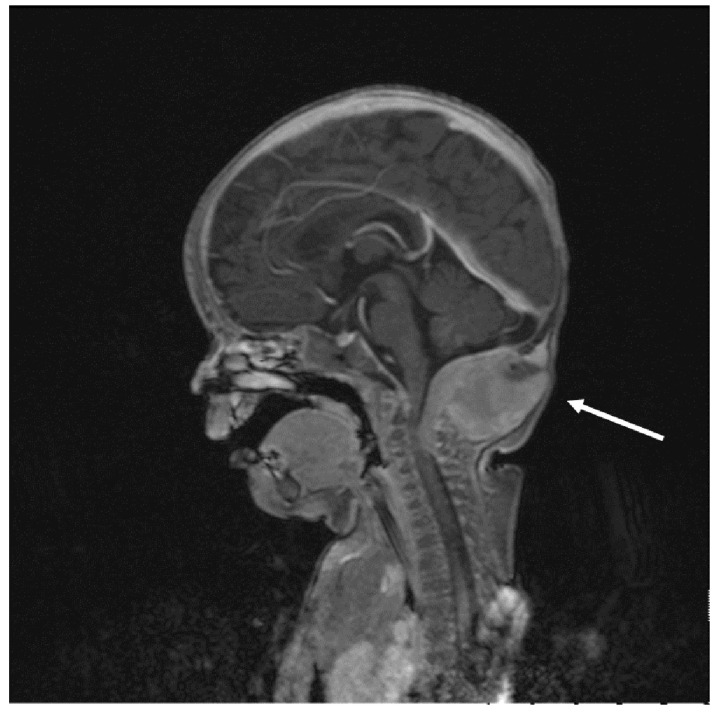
Patient 3: a sagittal T1-weighted MR scan shows large, suboccipital, mainly extracranial pathological solid mass compressing the spinal cord (C1–C3).
